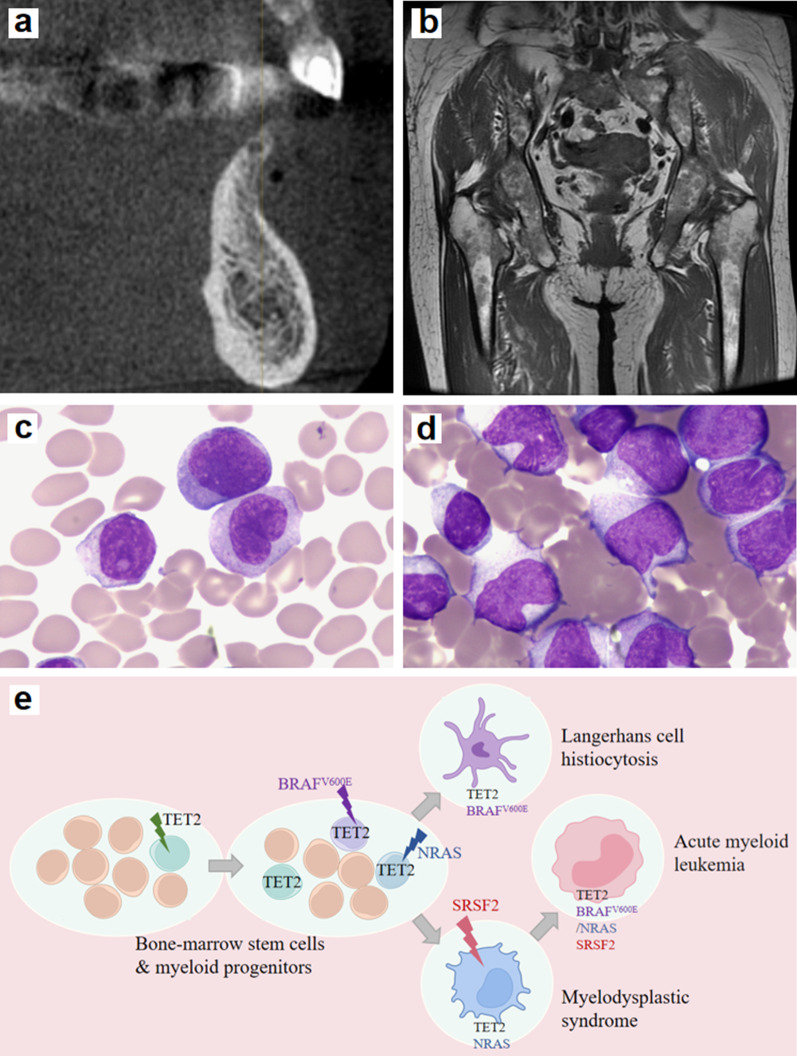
Imaging findings and cell morphology findings. a X-ray shows bone destruction. b MRI shows inhomogeneous signal intensity at pelvic bones, lumbosacral vertebrae and bilateral femurs. Cell morphology test shows myeloblasts and promonocytes in c the peripheral blood and d bone marrow increased. e The hypothesized order for the appearance of mutations
